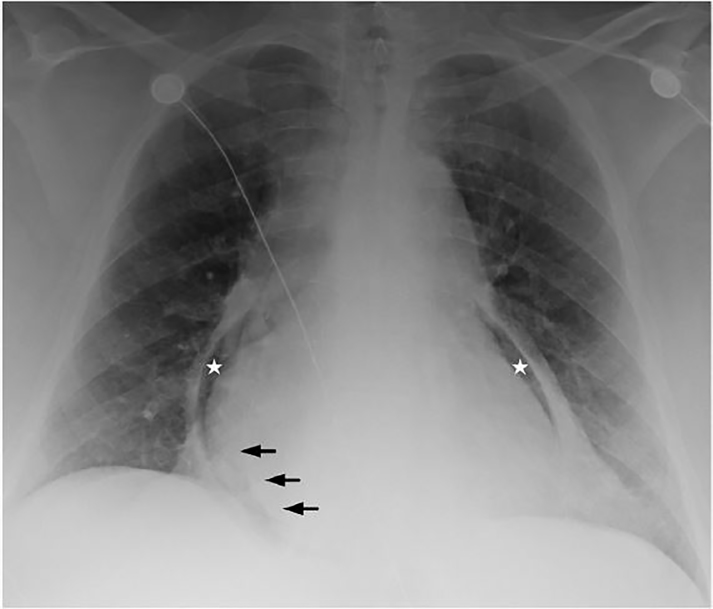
Portable anterior-posterior chest radiograph reveals pneumopericadium (stars) and dependent fluid (arrows) between myocardial and pericardial fat.
